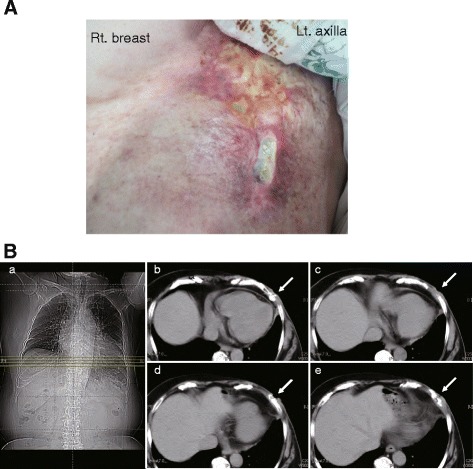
Gross appearance of the affected area and computed tomographic images of the left chest wall at the first visit. a A skin ulcer with redness and pus discharge was observed. b Computed tomographic images showing that the fistula did not connect into the thorax (*arrows*)
